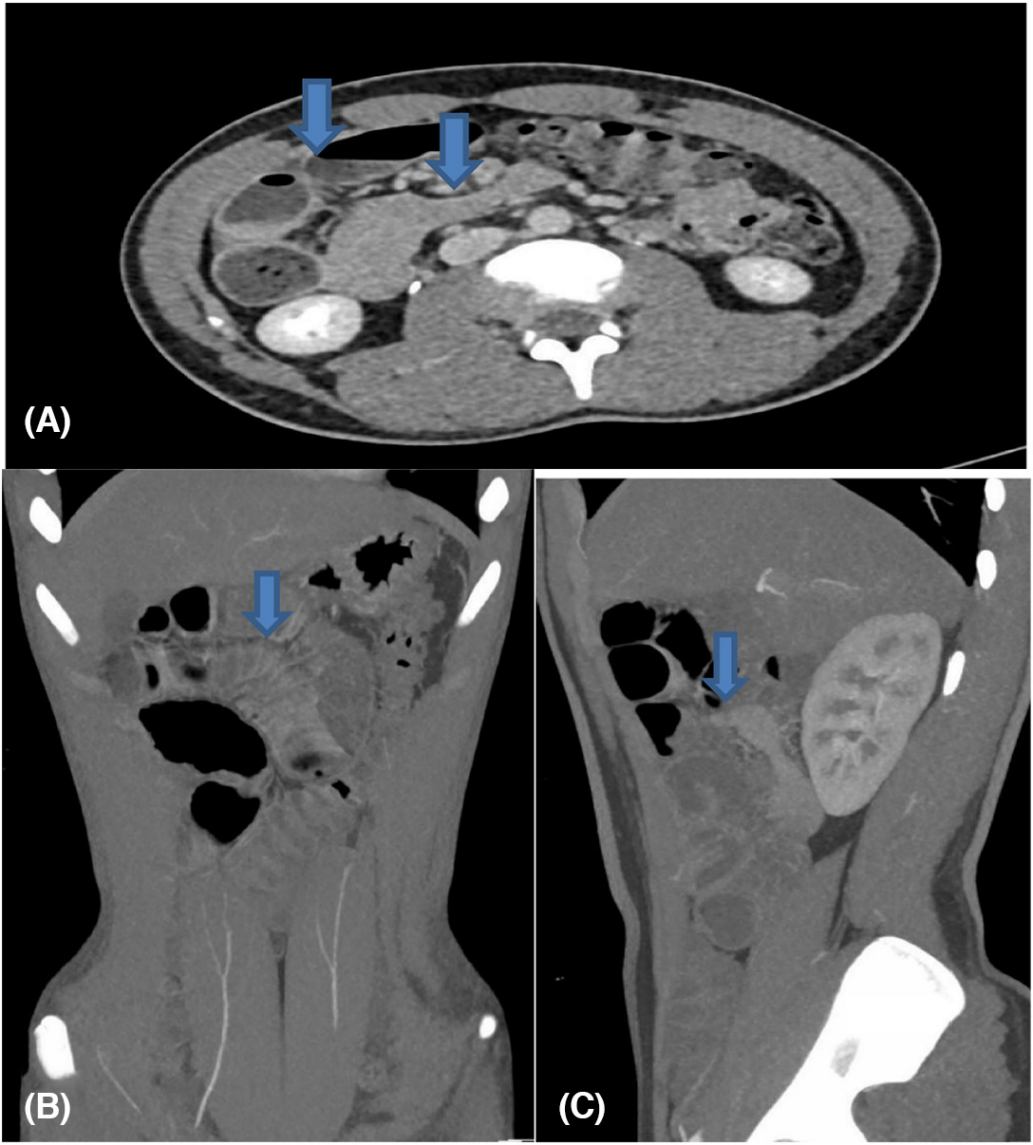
CT Scan abdomen showing findings of intestinal obstruction as mentioned earlier (A) axial view with arrows showed collapsed and distended small bowel (B) coronal view with arrow showing significant dilated jejunal loops and (C) sagittal view showing transitional area between dilated and collapsed small bowel.
